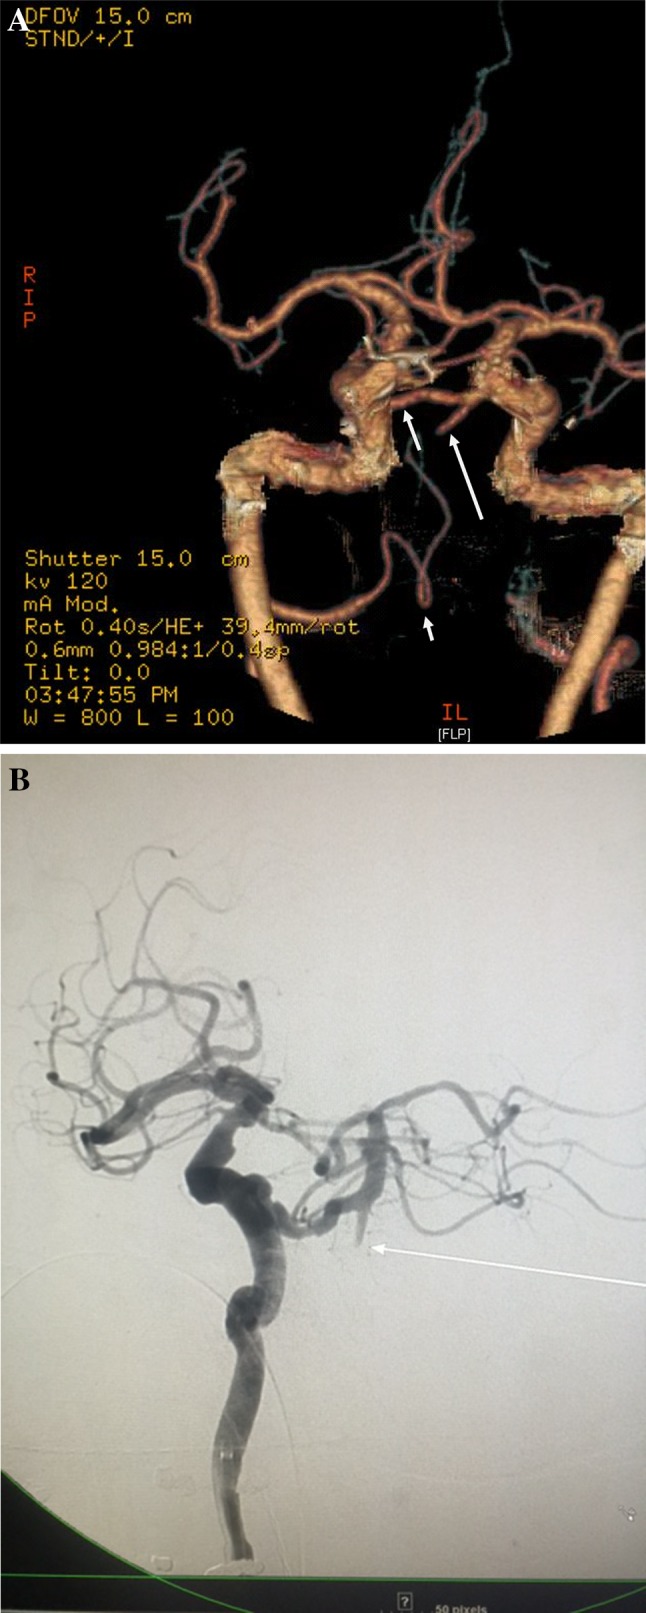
a CT angiogram reconstruction showing that the right vertebral artery terminates in the posterior inferior cerebellar artery (small arrow) that the distal basilar artery is supplied by a persistent trigeminal artery arising from the right internal carotid artery (midsize arrow) and that the stump of the distal left vertebral artery is just visible (large arrow). b Right internal carotid arteriogram showing the trigeminal artery supplying the distal basilar, arrow shows the stump of the occluded left vertebral artery
